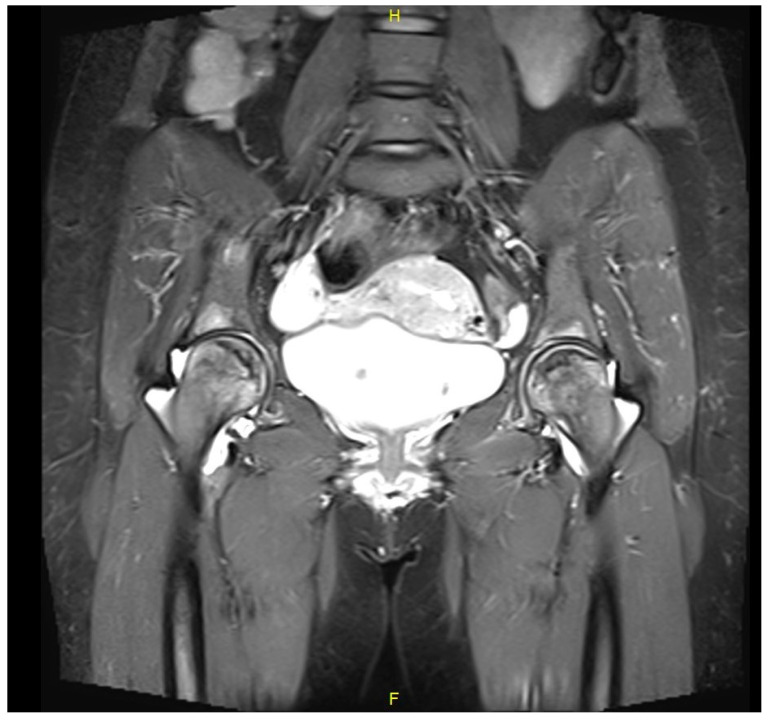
A coronal plane t-2 weighted MRI of pelvis and hip joints of Case 1 showing bilateral pre-collapse changes of femoral heads and joint effusions. These findings are compatible with simultaneous bilateral osteonecrosis.
